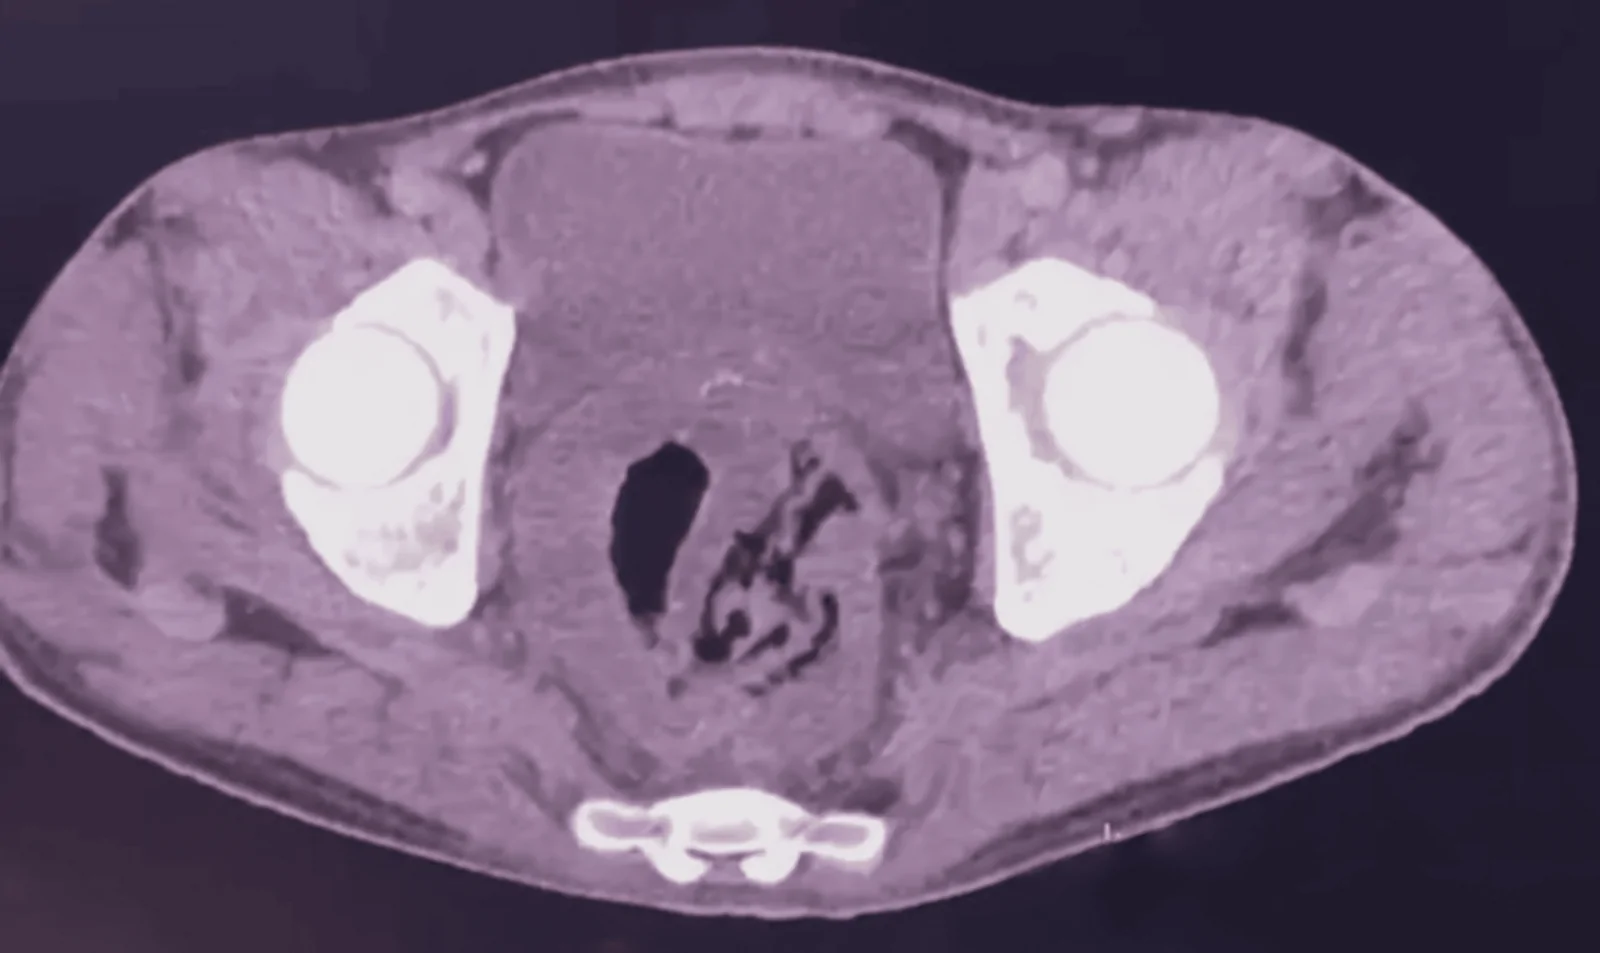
Axial abdominopelvic computed tomography image in Case 2. Axial abdominopelvic computed tomography image showing irregular circumferential thickening of the upper rectum and rectosigmoid junction with luminal narrowing.
